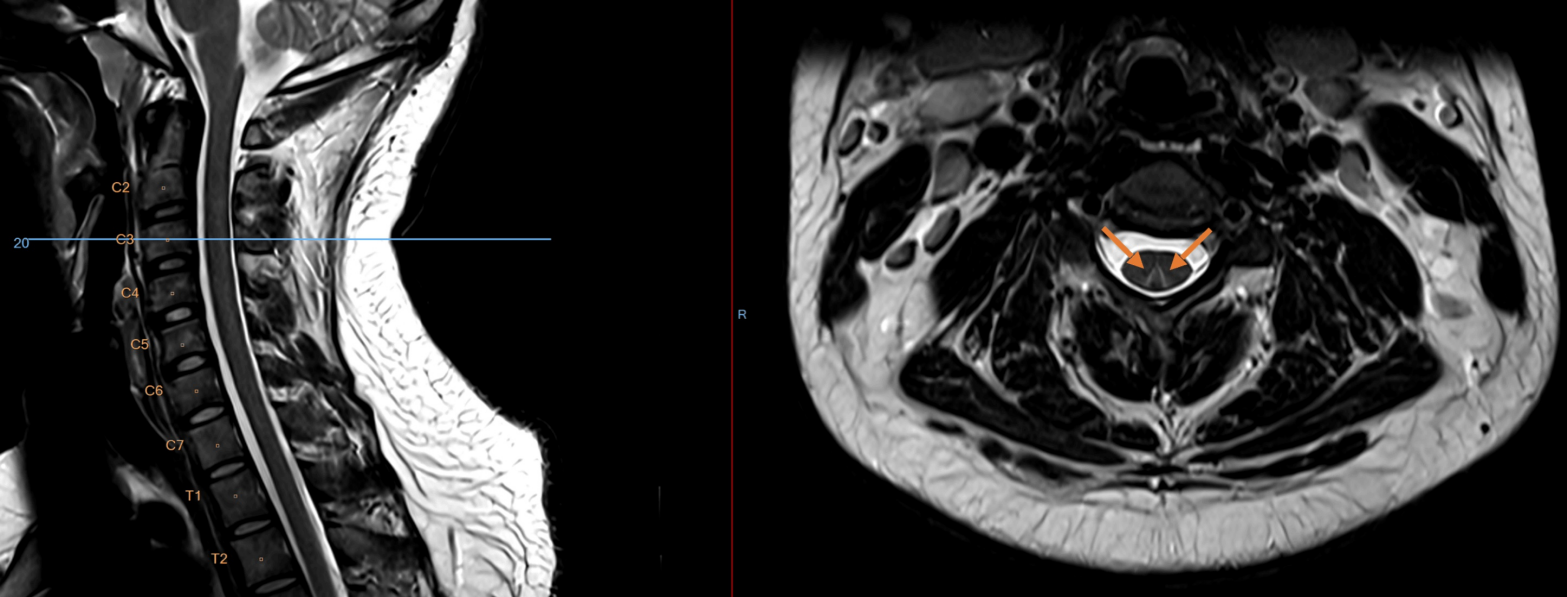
Axial T2-weighted MRI (3T) imaging of the cervical spine (C3)
